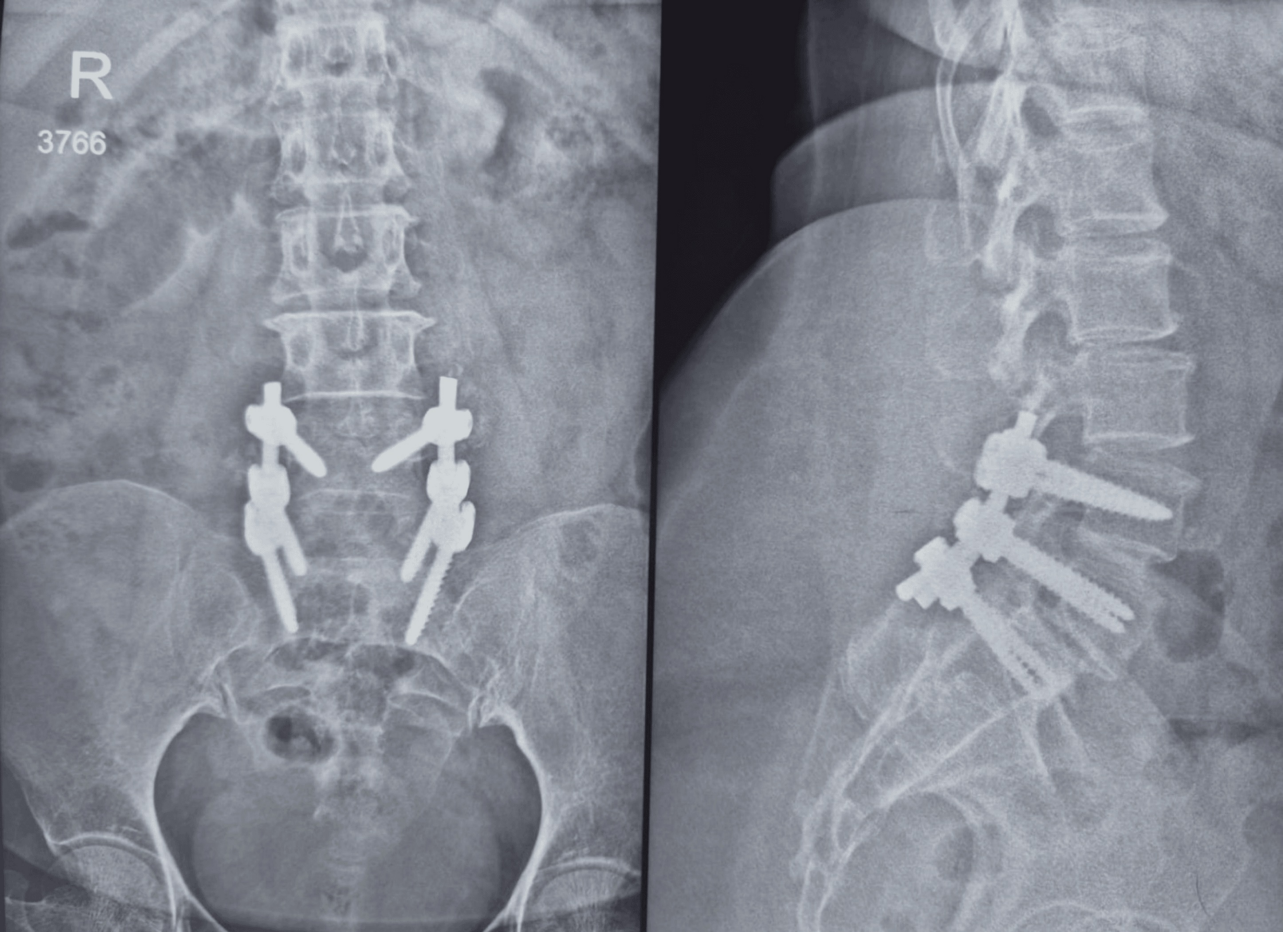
Six-month postoperative X-ray of the lumbosacral spine, showing anteroposterior and lateral views. Plain radiography of the lumbosacral spine showing anteroposterior and lateral views with three-level fixation of L5-S1 spondylolisthesis, including L4-L5 and L5-S1 pedicle screw fixation and posterolateral fusion, six months postoperatively, showing features of fusion.
